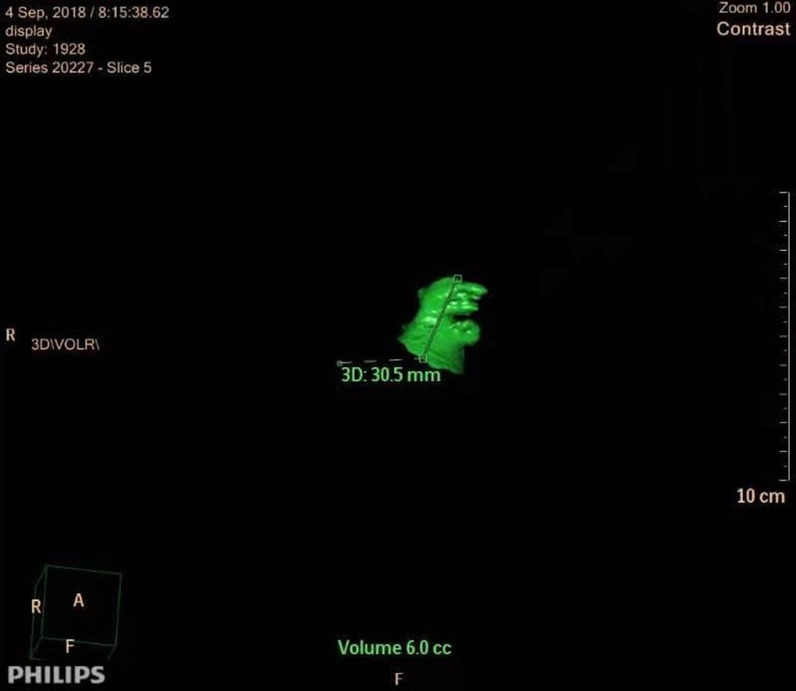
Three-dimensional volume of the left atrial appendage in CT
